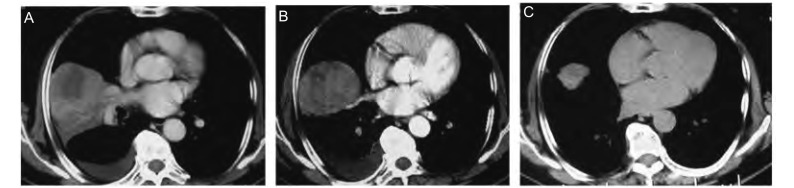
胸部CT显示右肺下叶肿块的改变。A：CT显示右下肺见一范围约8 cm×6 cm×6.2 cm的软组织肿块影（2009-05-08）；B：第一次化疗后肿块较前缩小，胸水减少（2009-06-10）；C：第三次化疗后肿块较前明显缩小，胸水消失（2009-08-11） The changes of chest CT scan. A: Chest CT scan shows a 8 cm×6 cm×6.2 cm lobulated mass in the lower right lobe (2009-05-08); B: The mass is become smaller after the first chemotherapy (2009-06-10); C: The mass is become smaller obviously and pleural effusions disappear after the third chemotherapy (2009-08-11)
